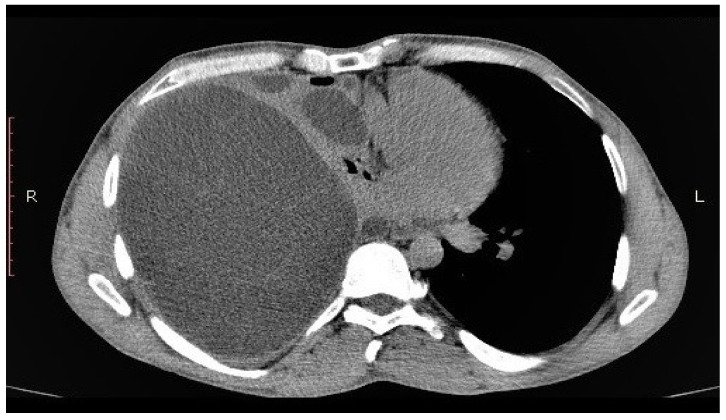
Computed tomography (CT) view of primary pleural hydatid disease.
